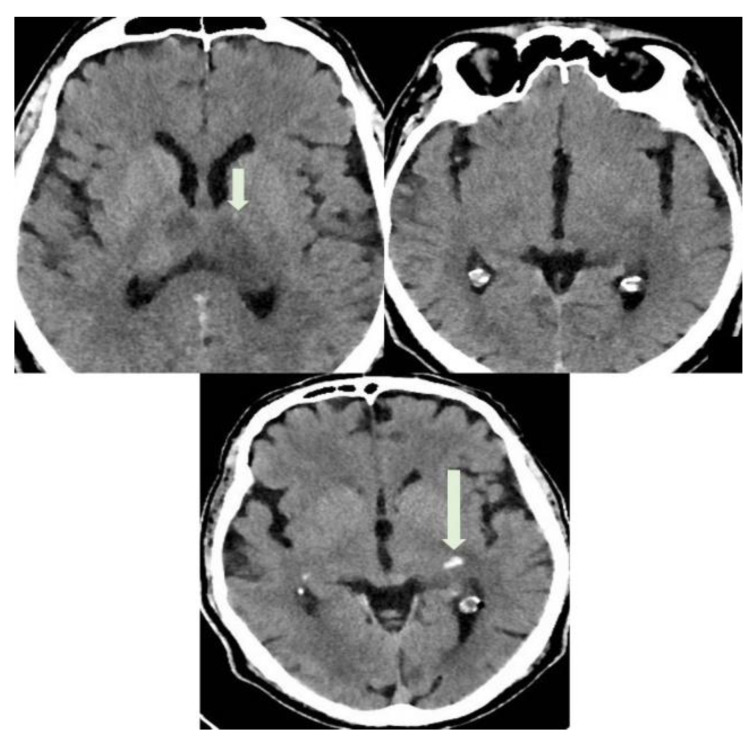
A CT with use of a medium contrast was performed the following day to better appreciate the vascular extension of the thrombosis. In the figure, axial CT images show hypodense areas in thalami and left parahippocampal region (short arrow, ischemic stroke lesions) and spontaneously hyperdense focus in left parahippocampal region (long arrow). This last was interpreted as a focal hemorrhagic transformation of the stroke. CVT in COVID-19 disease has been associated with a strong activation of the inflammatory chain. Specifically, it has been proven the implication of neutrophil extracellular traps (NETs), impaired systemic microcirculatory function, downregulation of natural anticoagulation phenomena and vascular endothelial dysfunction. Alveolar capillary microthrombi from COVID-19-induced immunothrombosis have been also described [3]. Other studies have reported CVT after vaccination against COVID-19 [6]. Thrombotic thrombocytopenia and cerebral venous sinus thrombosis have been observed in patients after receiving the adenovirus-based vaccine (AstraZeneca) and after the first dose of the mRNA-based vaccine, as in our case. Of note, a pathological mechanism which causes CVT in these cases has been postulated, known as “vaccine-induced immune thrombotic thrombocytopenia” (VITT) [7]. Low platelet count and high D-dimer values, as in our case, can assist the diagnosis of VITT after vaccination [8].
